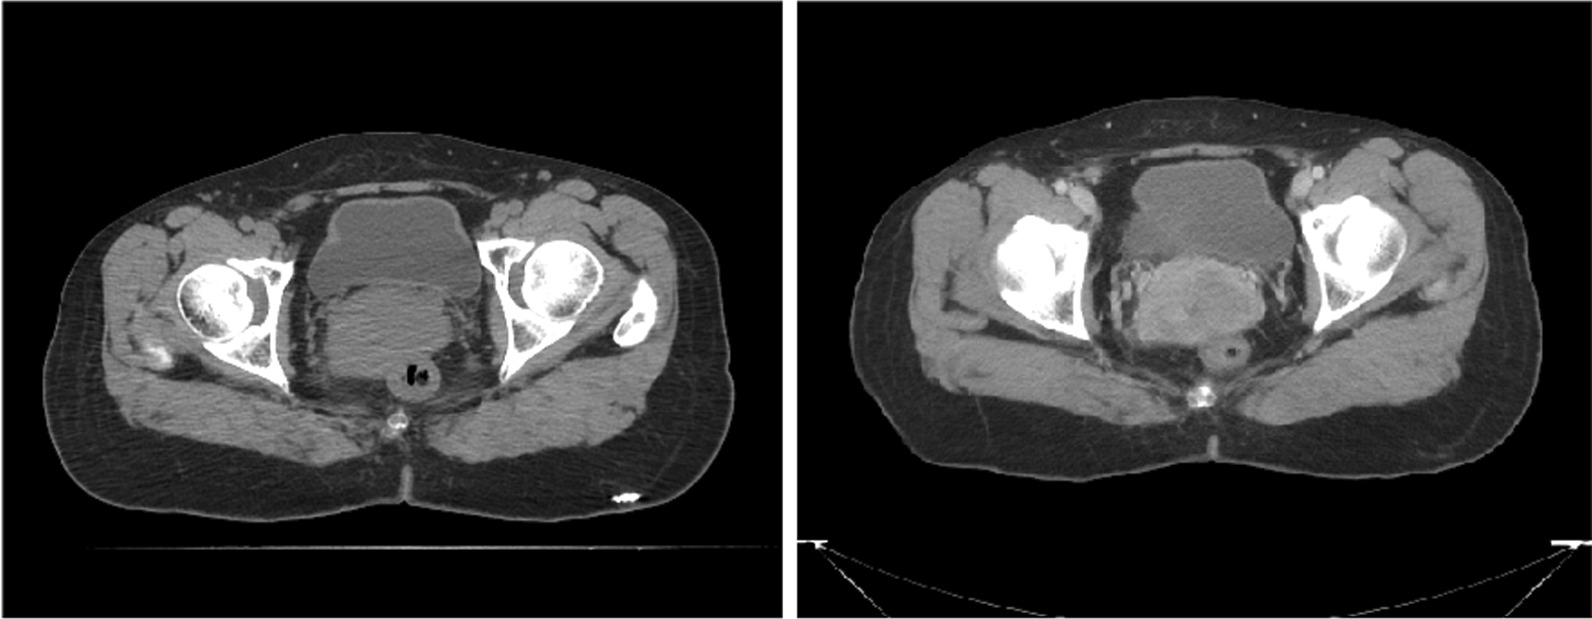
Difference of plain CT (left) and contrast-enhanced CT (right)
